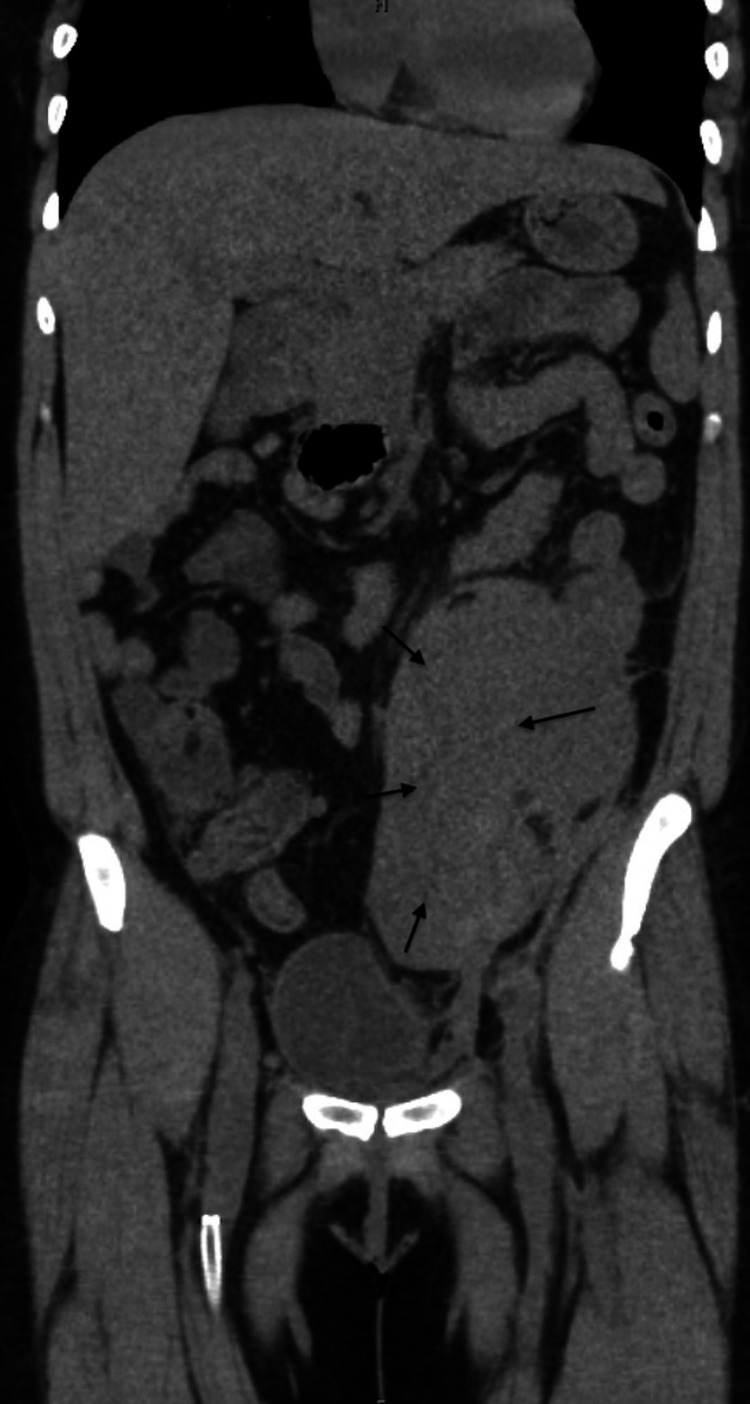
CT coronal image Retroperitoneal hematoma "arrows"
